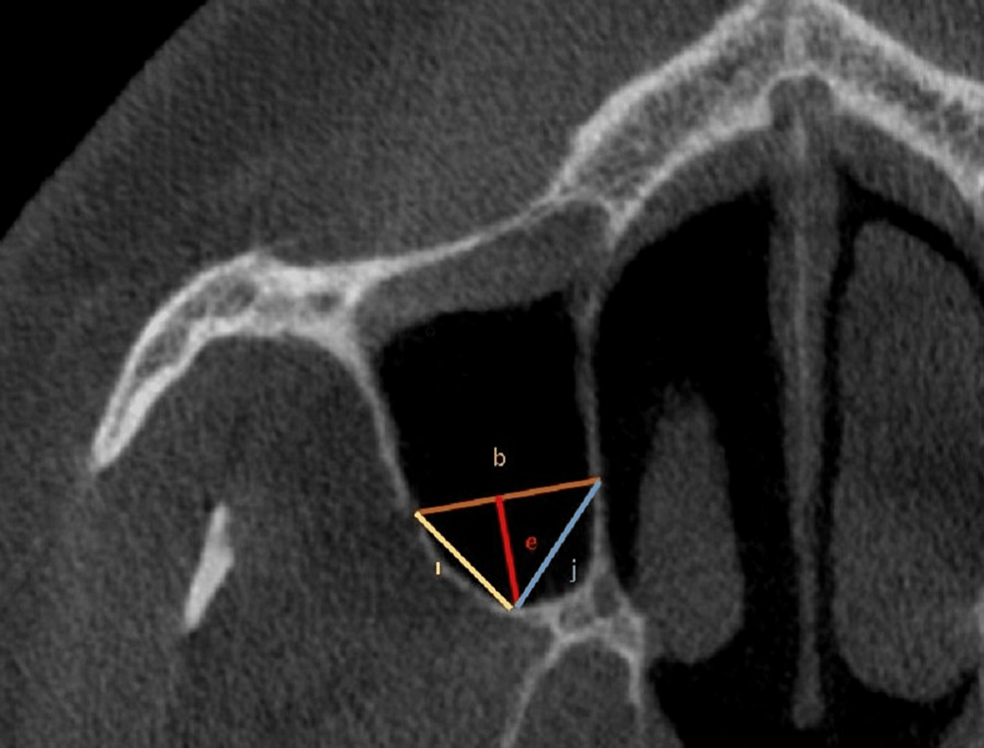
Other measurements made on the CBCT. b = maxillary sinus width; e = distance from the AAA to the sinus floor; i = oblique distance from the AAA to the sinus floor; j = distance from the sinus side wall to the floor. AAA = alveolar antral artery; CBCT = cone-beam computed tomography
